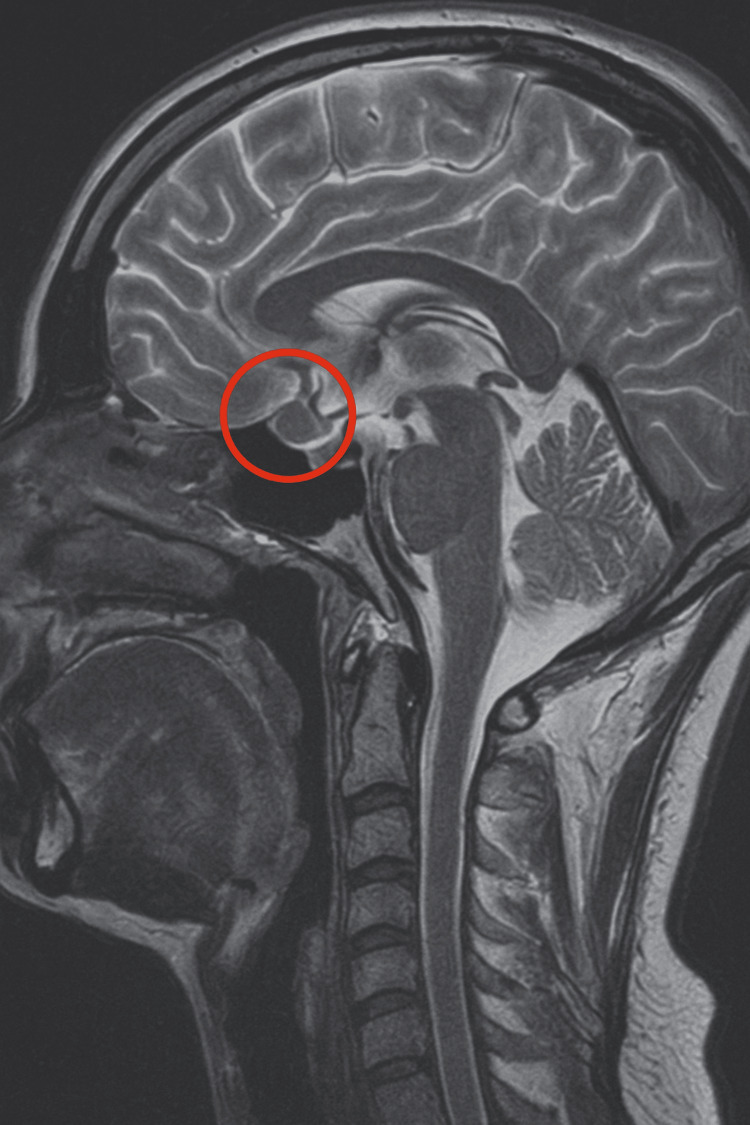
T1-weighted sagittal MRI scan showing a corticotroph pituitary microadenoma (4 × 3 mm) circled in red The lesion is localized within the anterior pituitary gland, consistent with an ACTH-secreting adenoma causing Cushing’s disease in the patient. MRI, magnetic resonance imaging; ACTH, adrenocorticotropic hormone
